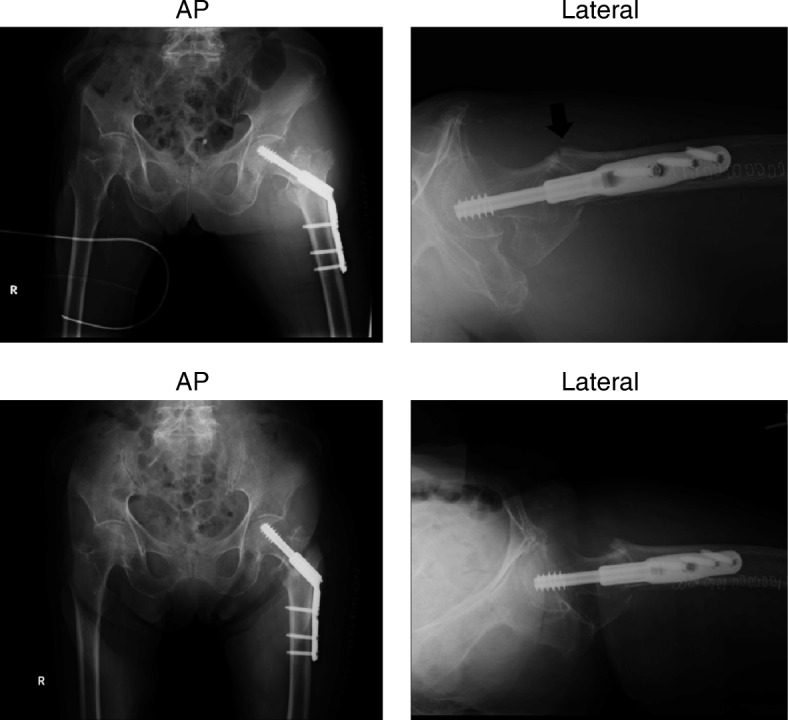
Postoperative displacement of trochanteric fractures. The upper section shows the postoperative radiograph, confirming reduction to the neutral fracture alignment. The lower section shows a radiograph, obtained on the postoperative day 14, confirming the negative fracture alignment. The black arrow indicates an angulation deformity at the fracture site
